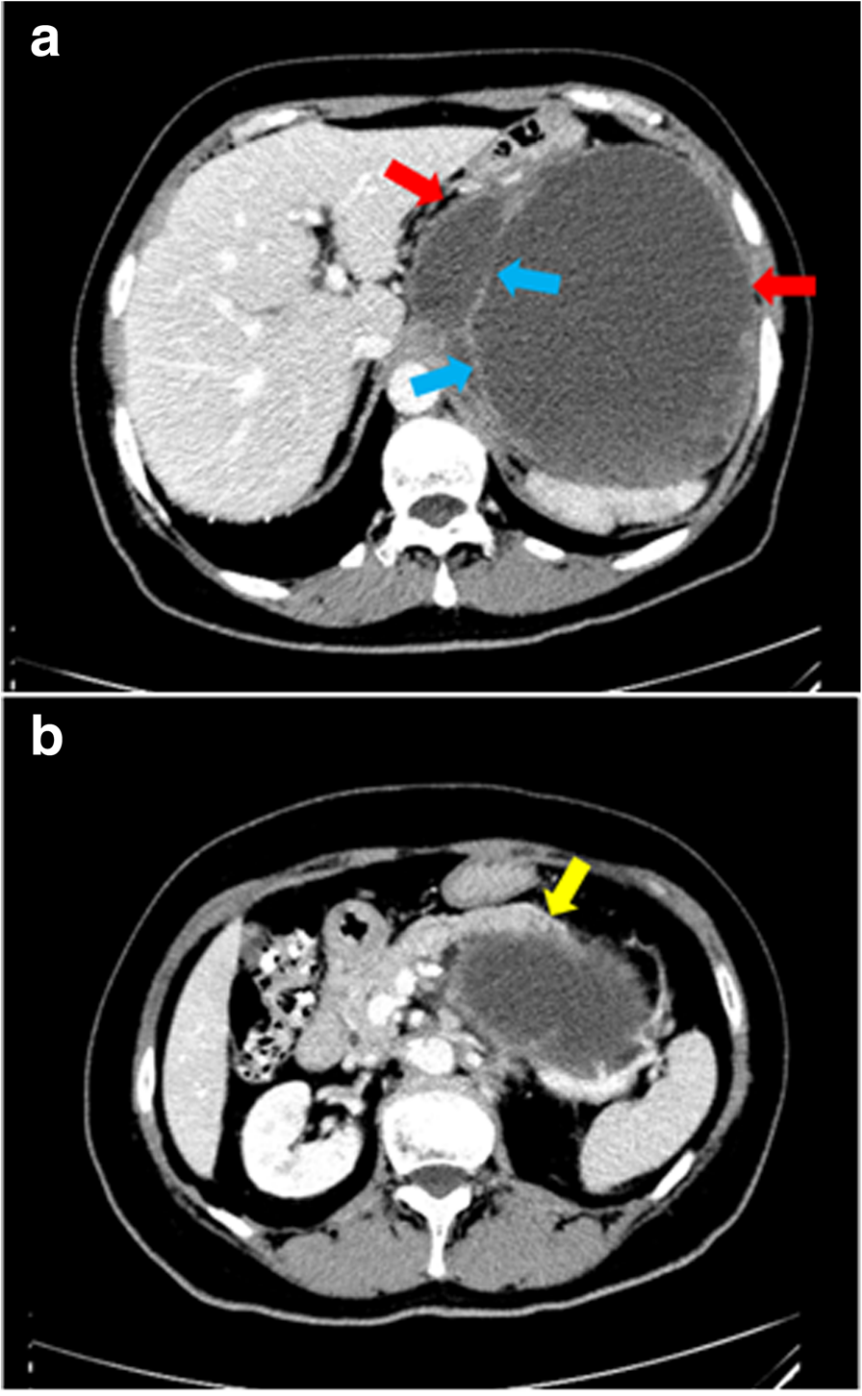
Abdominal CT demonstrates a cystic hypointense shadow in the upper left abdomen. a Septations (blue arrow) are seen in the tumor (red arrow), which is approximately 15.6 cm × 14.7 cm × 13.6 cm in size. b The pancreatic gland (yellow arrow) is compressed and displaced anteriorly, and the border between the pancreatic tail (white arrow) and the tumor is indistinct
